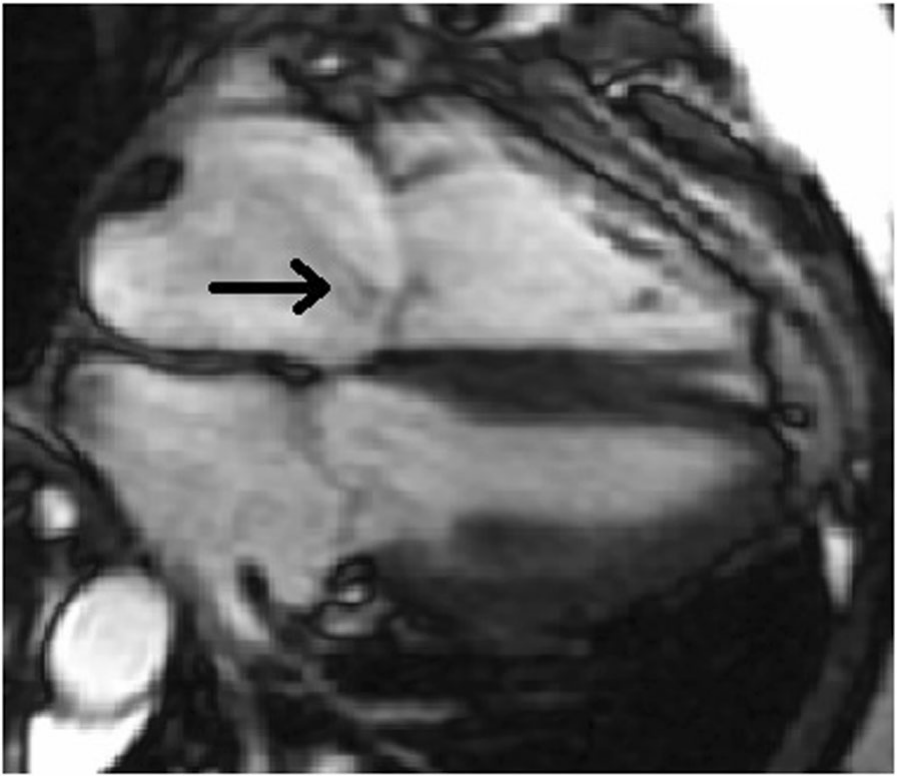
Tricuspid regurgitation in a dilated right ventricle and right atrium. Fiesta cine sequence MRI image demonstrates a four-chamber view with incomplete closure of the tricuspid valve due to right ventricular dilatation resulting in tricuspid valve regurgitation (arrow)
